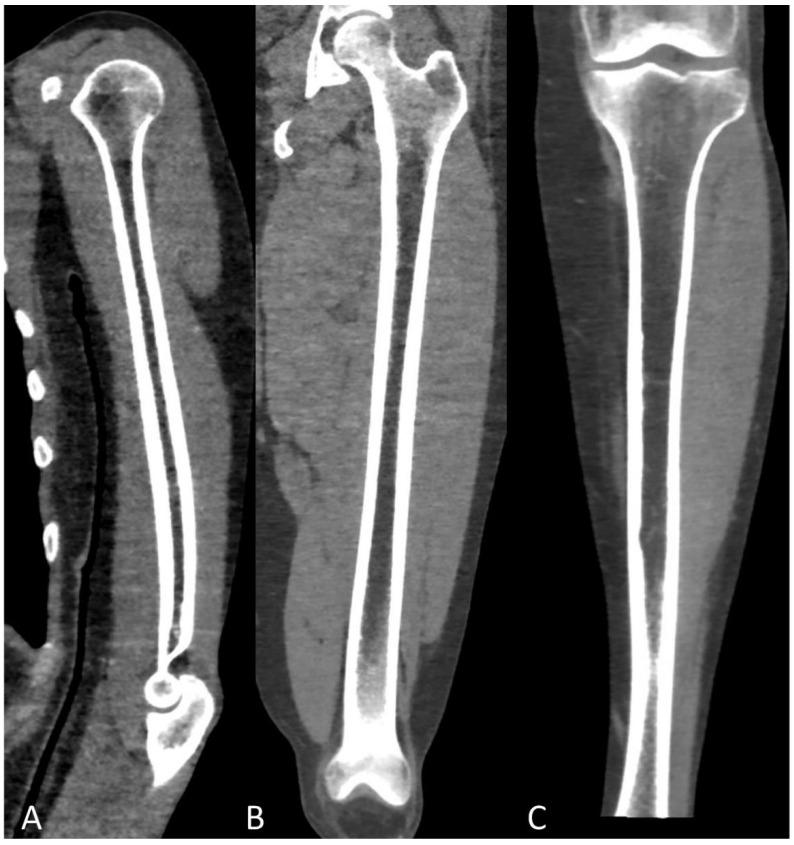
Multiplanar CT images with “soft tissue” window showing the distinctive aspect of normal (A) humeral, (B) femoral, and (C) tibial fatty bone marrow on low-dose CT in healthy adults.
